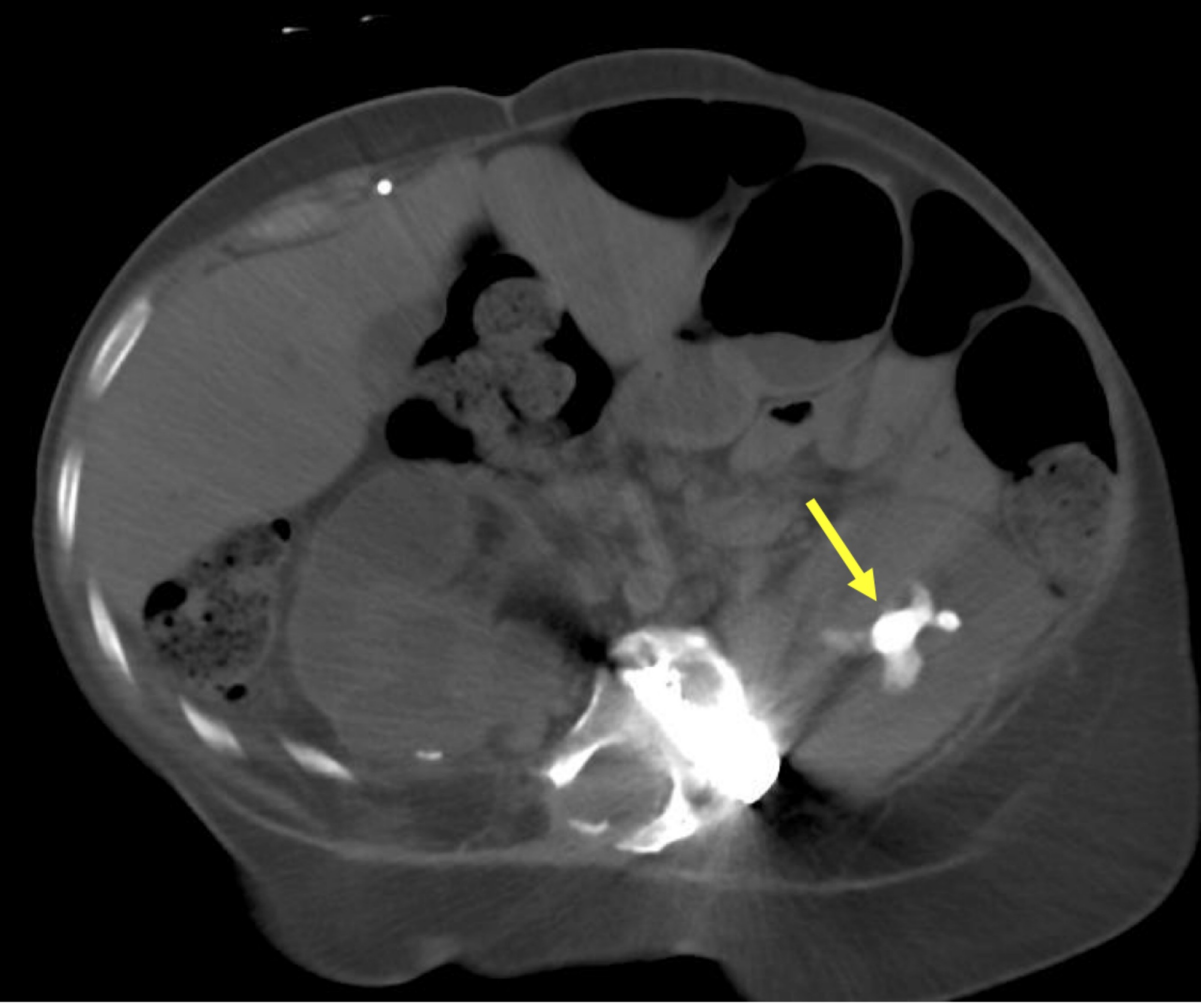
A non-contrast axial computed tomography image showing a large staghorn calculus (yellow arrow)
